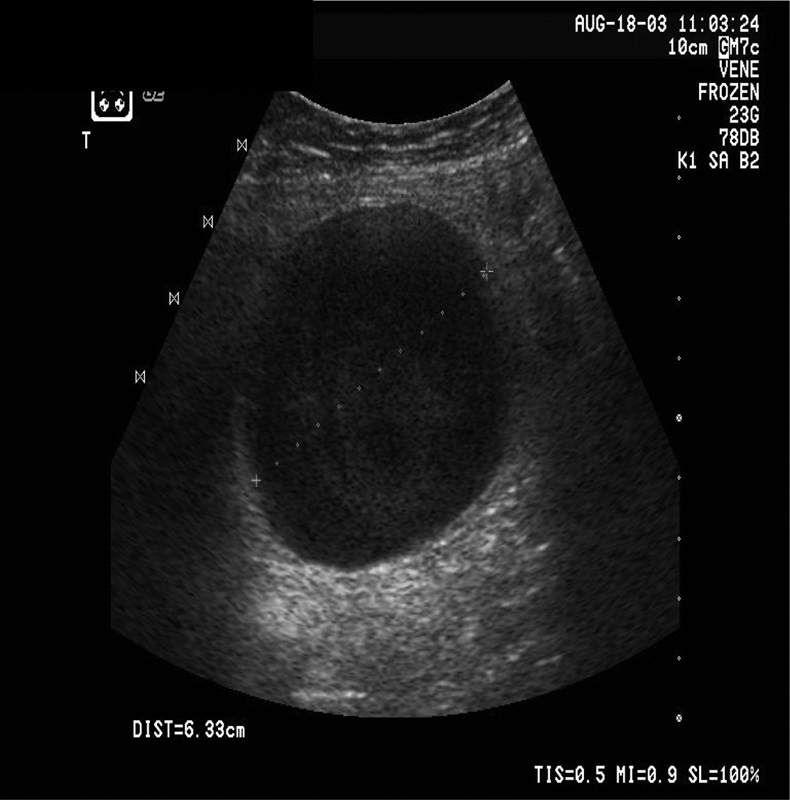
Under ultrasound examination, an echo-poor formation can be identified as a vessel.
